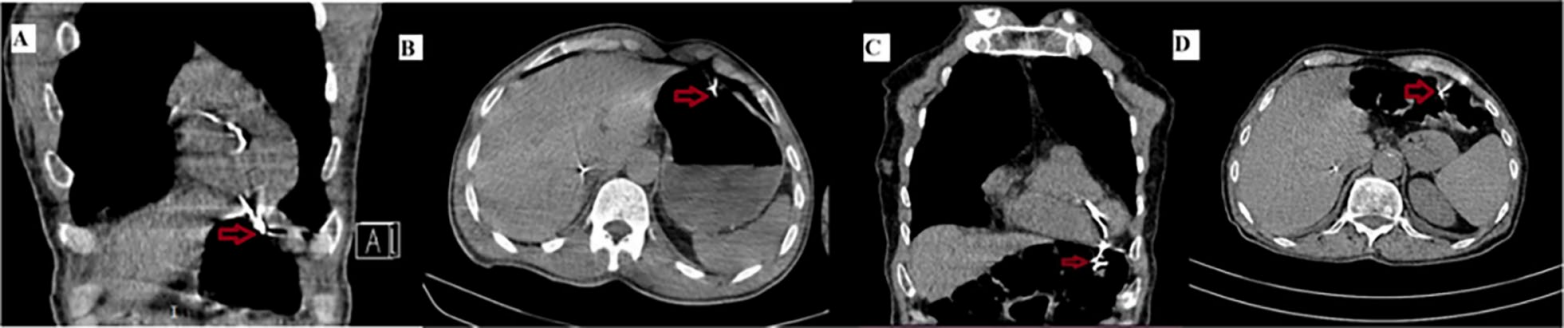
Coronal (A) and transverse section (B) CT images of one patient showing temporary lead perforating RV, diaphragm and fundus of stomach(arrows). Coronal (C) and transverse section (D) CT images of another patient showing temporary lead perforating RV, diaphragm and transverse colon (arrows)
